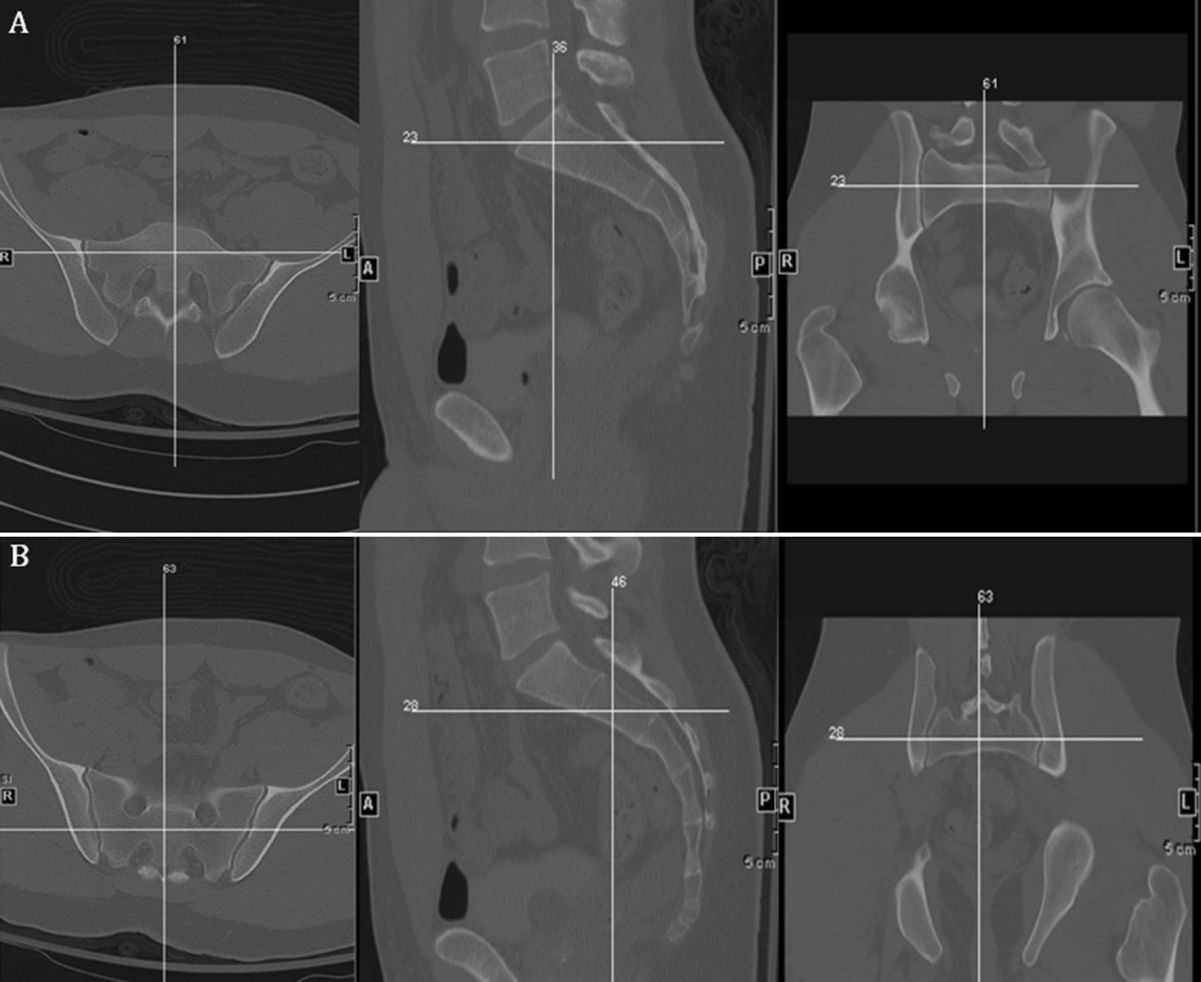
CT images depicting the cross-referencing technique used for localizing the mid body point of S1 (a) and S2 (b) for measurement in axial, sagittal and coronal reconstructions
